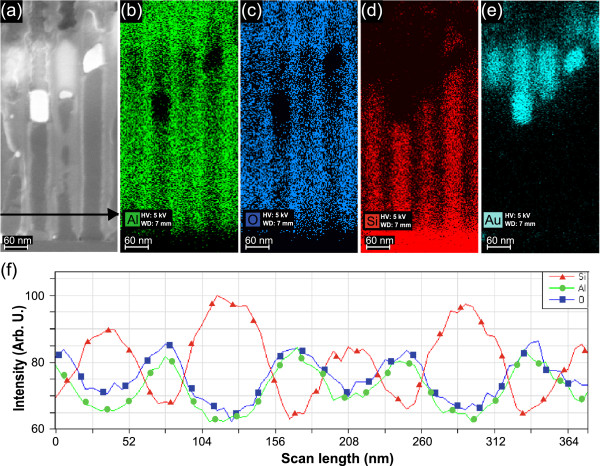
Energy dispersive X-ray (EDX) analysis of Si NW. (a) SEM image of the cross section, (b) aluminium cartography, (c) oxygen cartography, (d) silicon cartography, (e) gold cartography and (f) profile counts of oxygen, aluminium and silicon, along the arrow of (a). The EDX analyses were conducted at 5-kV high voltage and for a 7-mm WD.
